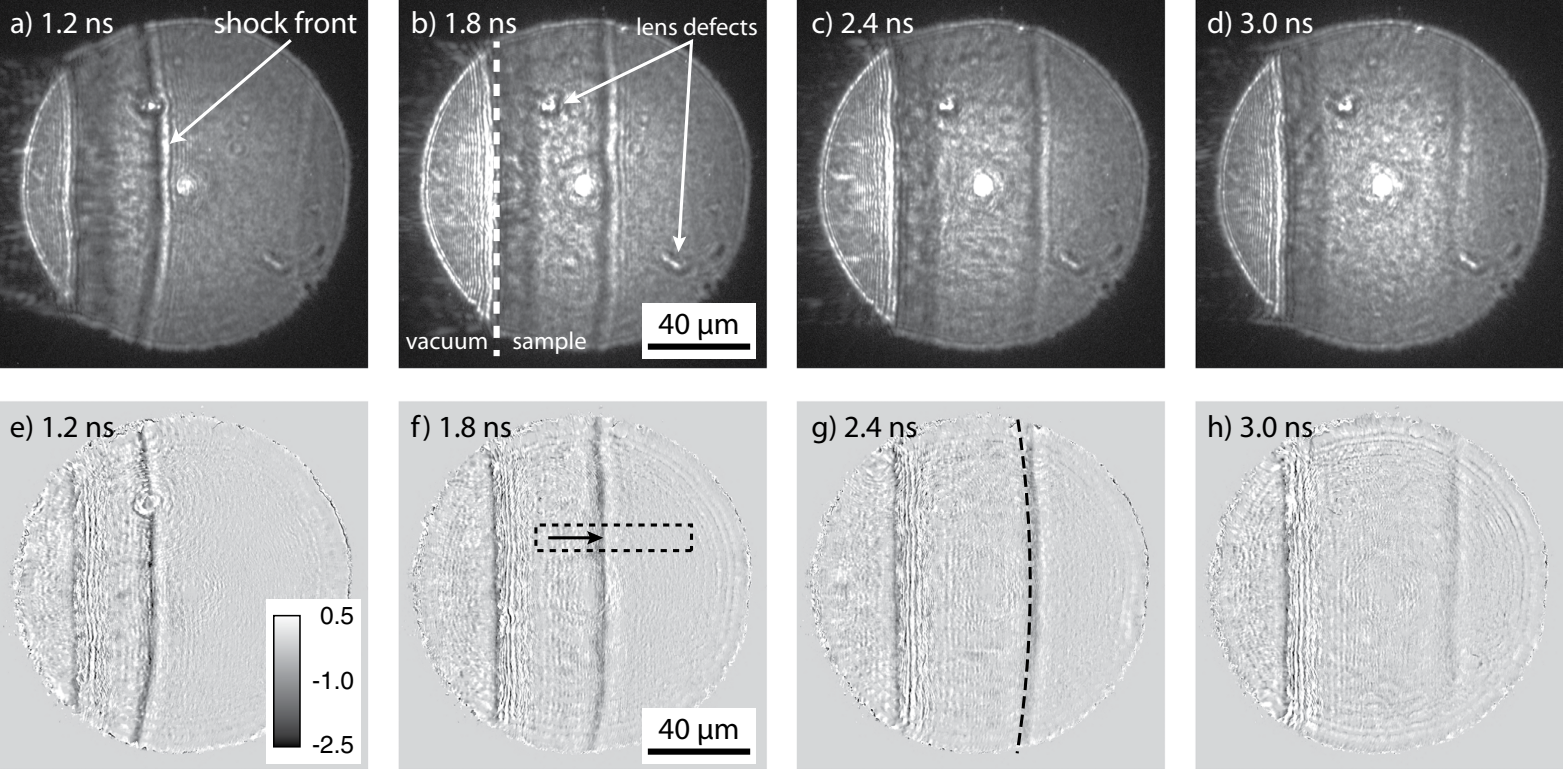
(a–d) Phase-contrast images measured with a high-resolution x-ray detector at a distance of 4214 mm behind the sample. Specific time delays are indicated in each image. (e–h) Corresponding phase maps obtained by iterative phase retrieval from the images above. In order to enhance the visibility of shock-related features, the phase map obtained from just the sample without shock wave was subtracted from the phase map with shock wave. Gray values indicate the phase shift in radians [cf. inset in Fig. e)]. In Fig. f) a rectangular box highlights the area used to quantitatively determine the compression of the material.
